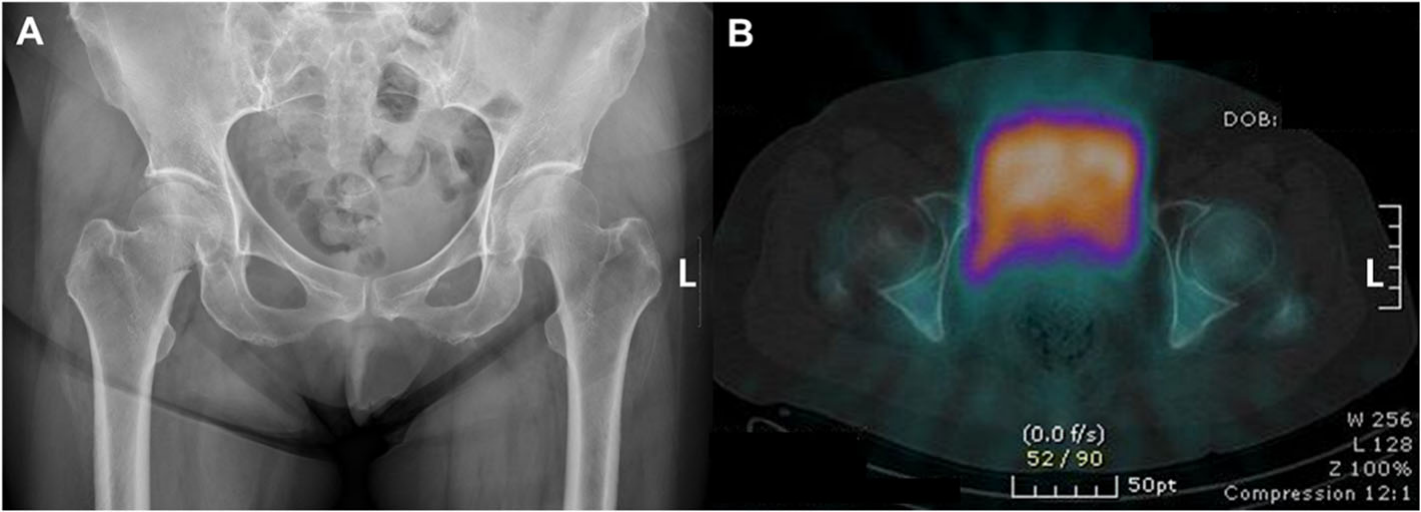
Qualitative analysis of SPECT/CT. a Right femur neck fracture is diagnosed by simple radiograph. b Photon uptake of the right femur head is decreased compared to the left femur head
